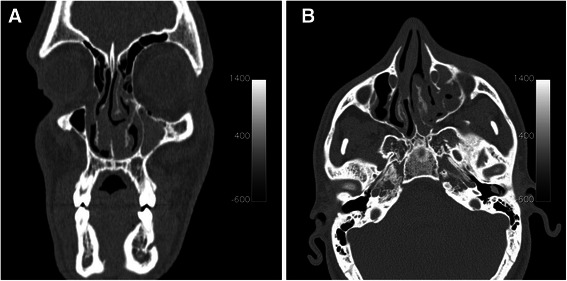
a–b Computed tomography image obtained 3 months after surgery. The reconstructed orbital floor and resolution of the left hemosinus are observed
